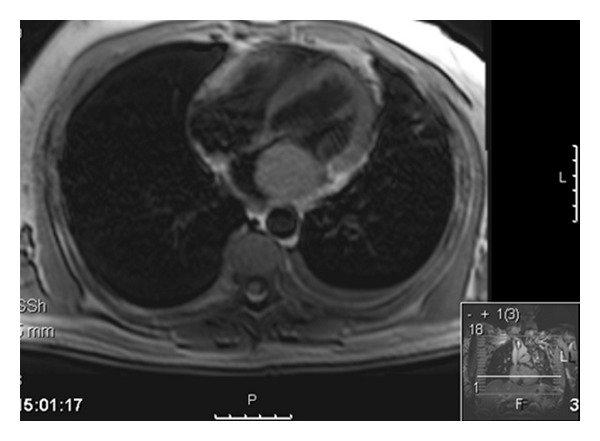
Cardiac magnetic resonance scan (MRI) showing left atrial mass obstructing left ventricular outlet tract.
